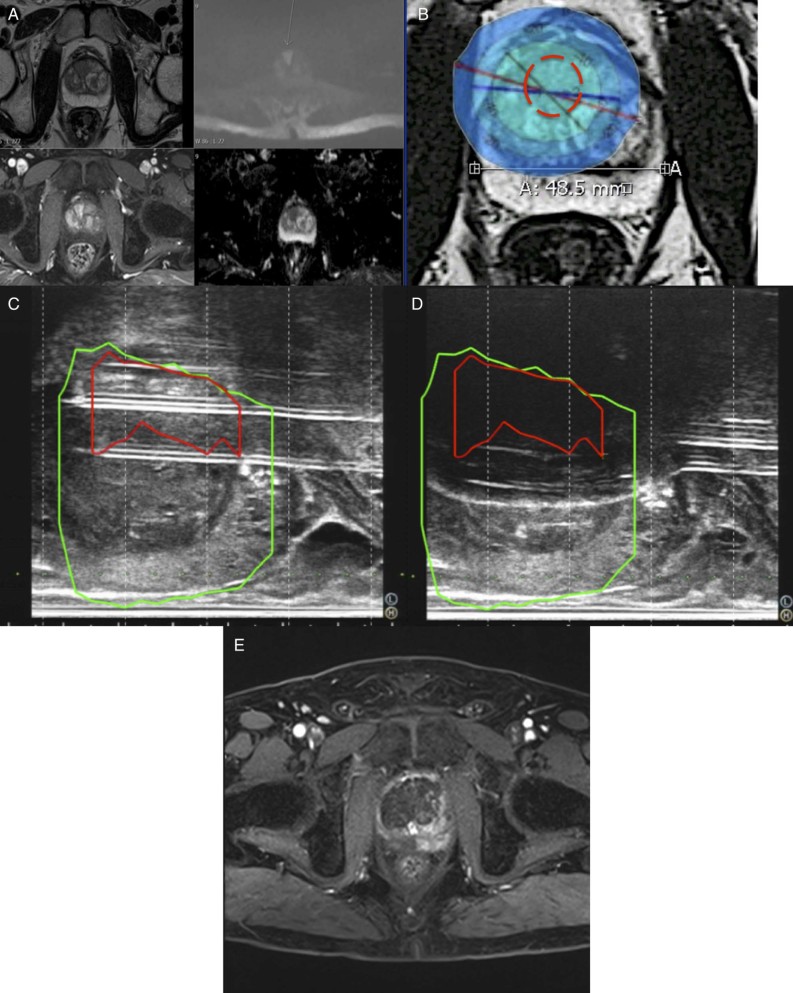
(A) Multi-parametric magnetic resonance imaging showing 18 mm max. diameter anterior prostate cancer. (B) Overlaid ice ball data, 1 cm apart at a duty cycle of 100% showing adequate coverage by −40°C isotherm over lesion. (C) Intra-operative needle placement using Biojet ultrasound (US) fusion system. (D) Intra-operative ice ball expansion. (E) Dynamic contrast enhanced magnetic resonance (MR) images showing area of necrosis and concordance with preoperative planning. (Color version available online.)
